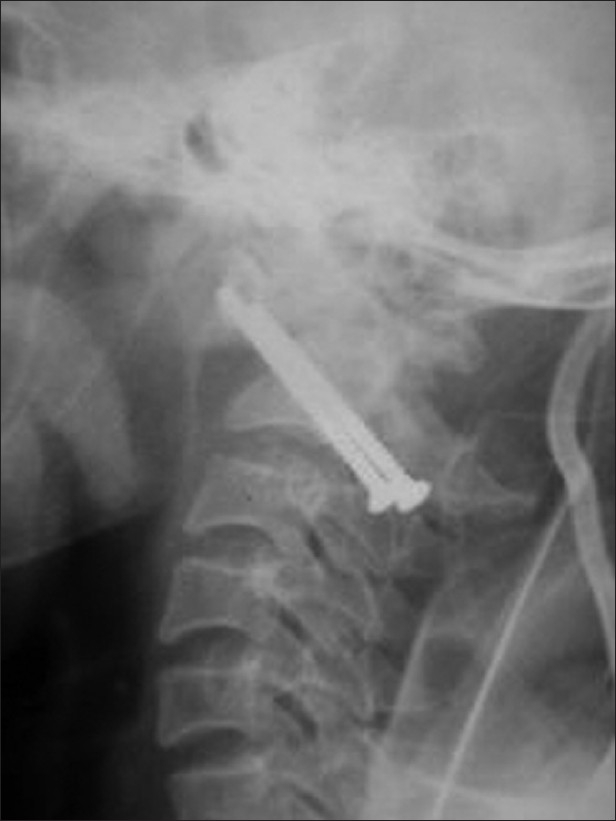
X-ray cervical spine lateral view showing transarticular C1 C2 fixation. The suboptimal reduction cannot be corrected after passing of the transarticular screw unlike in the lateral mass fixation where further reduction can be achieved by tightening the screws over the rods
